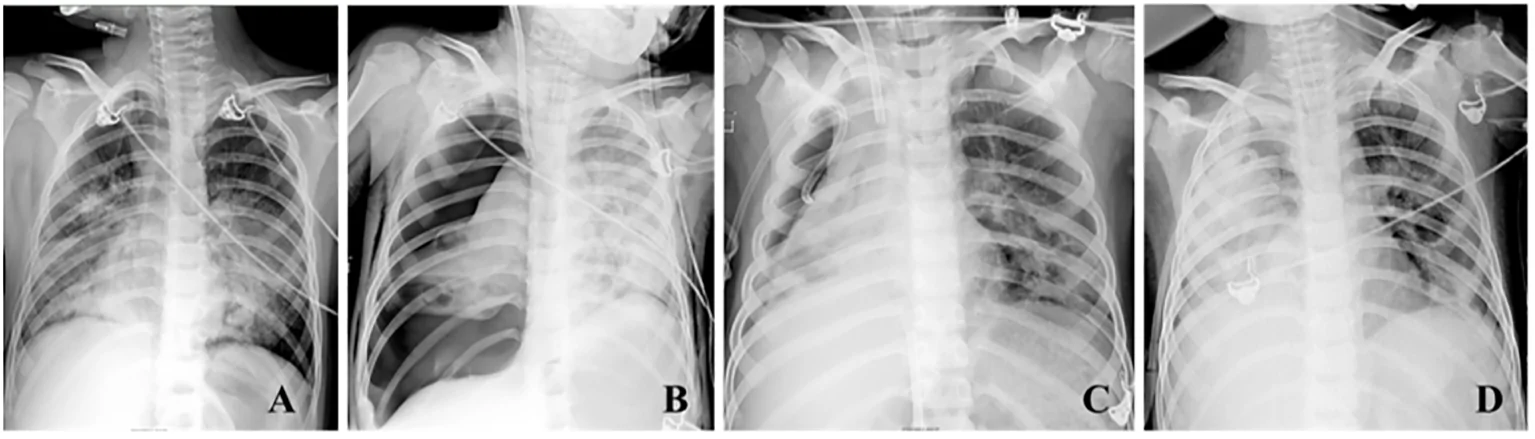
X-ray of the patient. (A) Bilateral patchy opacities, suggestive of pneumonia and potential pulmonary hemorrhage (day of admission). (B) Right-sided pneumothorax and bilateral patchy opacities with interval worsening (the 10th day of admission). (C) Right-sided pneumothorax showed partial resolution, with the appearance of a right pleural effusion; the left-sided patchy opacities had diminished, whereas the number of air-containing cystic lesions had increased (the 29th day of admission). (D) Right lung exhibited decreased aeration with patchy densities and cystic lesions; the left lung demonstrated increased and blurred bronchovascular markings with patchy infiltrates, which had progressed locally, while the air-containing cystic lesions remained largely unchanged (the 38th day of admission).
